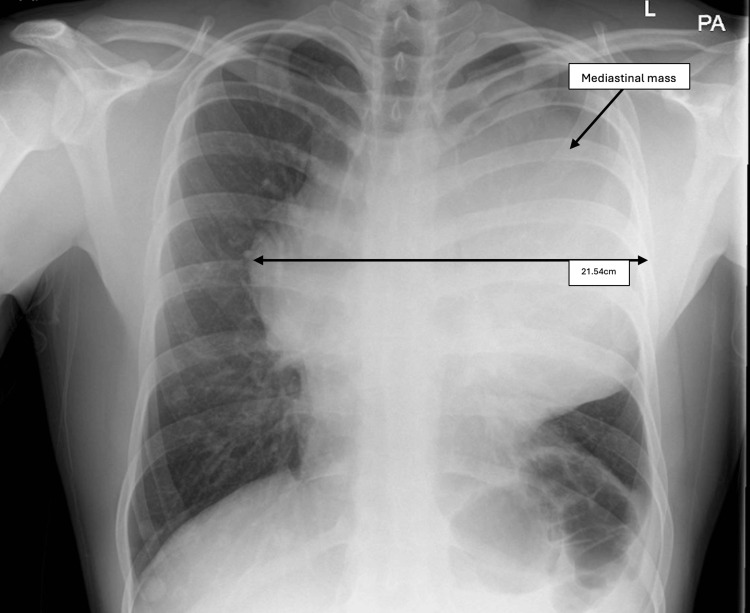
Posteroanterior chest radiograph demonstrating marked widening of the upper mediastinal contours with a large homogeneous opacity projected over the left upper and mid zones The black arrow indicates the superior aspect of the mediastinal mass. The double-headed arrow denotes the transverse diameter of the mediastinal mass. The appearances prompted urgent cross-sectional imaging for further characterisation.
